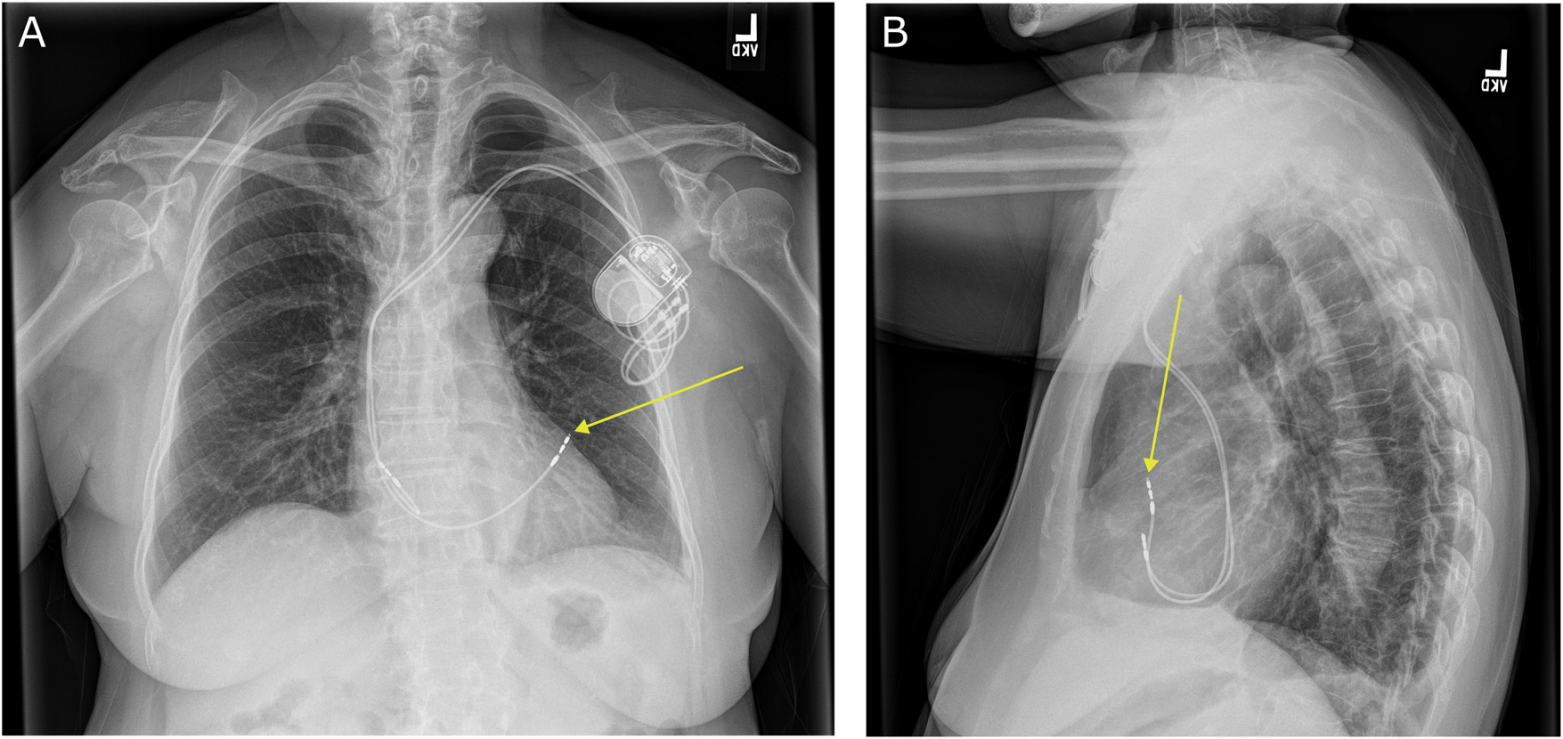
Posteroanterior (A) and lateral (B) radiograph of the chest. The dual chamber pacemaker generator is seen in the left upper chest wall. Pacemaker leads are intact. The atrial lead appears to be in placed in the right atrium. On frontal posteroanterior projection the tip of the right ventricular lead appears to project slightly beyond the cardiac border which is not evident on lateral view (yellow arrow). Small left apical pneumothorax is present.
